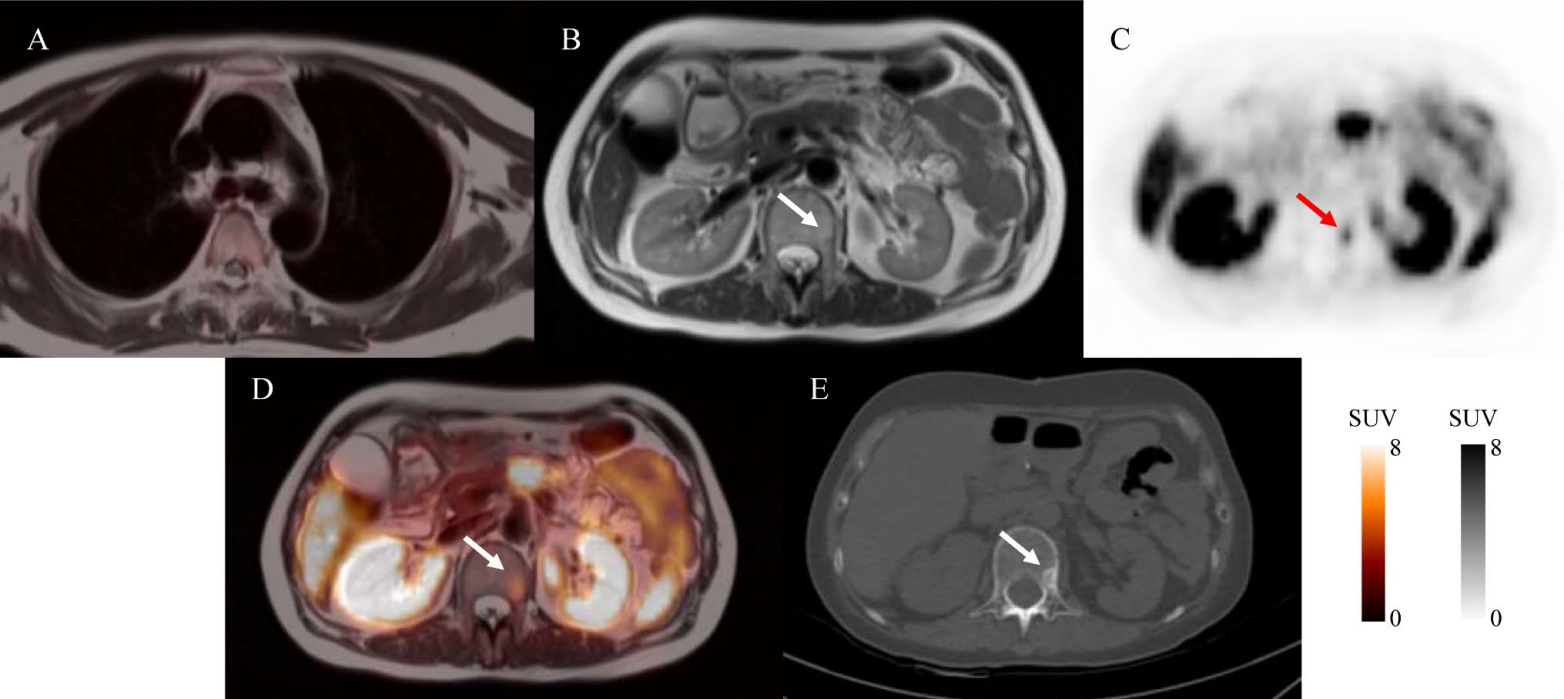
PET/MR-fused images showing blood pool uptake a SUVmax of 0.7 (A). Axial T2 weighted MR (B), axial ^68^Ga-DOTATATE PET (C), and axial ^68^Ga-DOTATATE PET/MR (D) images through the pelvis revealing a hypointense mass, DOTATATE-avid uptake in the L1 vertebrae (arrows, SUVmax:5.8). Follow-up CT image performed 22 months after initial imaging (E) revealed a pronounced new osteogenic L1 vertebral lesion (arrows) consistent with NET bone metastasis
